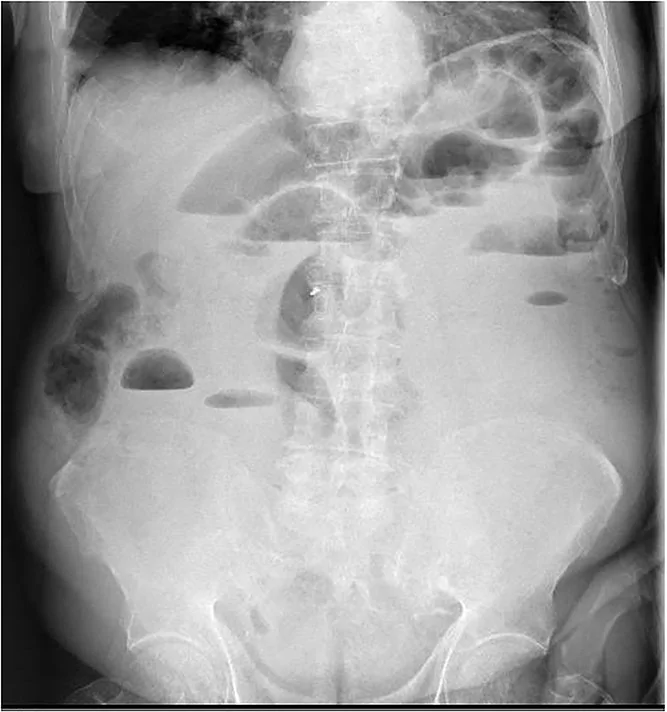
Upright plain abdominal radiograph showing distended small-bowel loops with multiple stepped air–fluid levels, consistent with intestinal obstruction.
